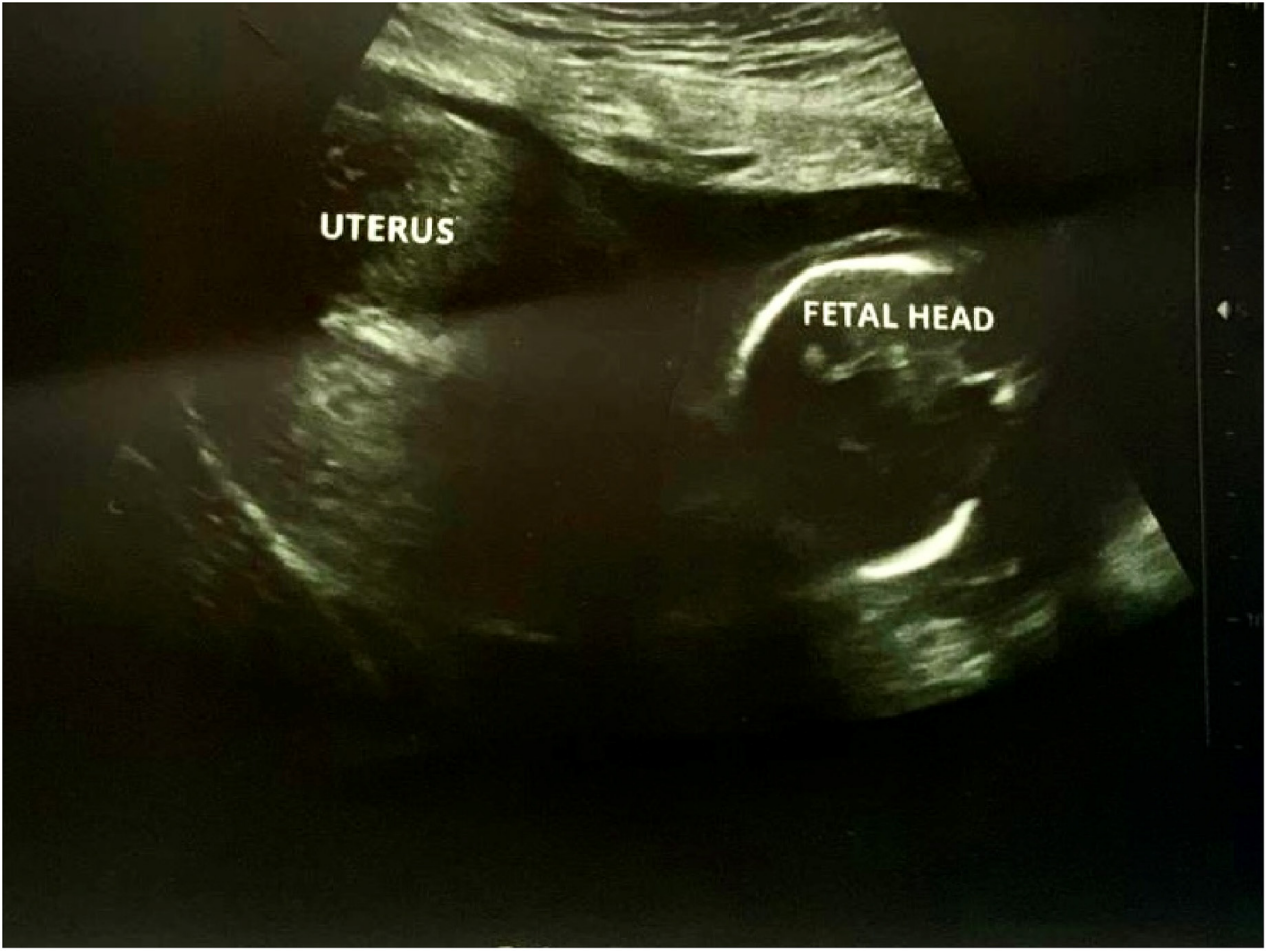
Ultrasound scan of the uterus showing the fetal head outside the uterine cavity.
